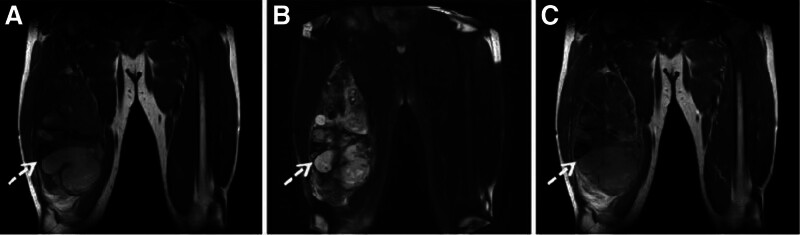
MRI revealed a huge space-occupying lesion with cystic mixed components, in the middle of the right thigh region with irregular shape and a poorly defined local boundary, measured 267 × 119 × 102 mm (white dashed arrow; A, T1-weighted coronal: T1 hypointense mass located over the middle of the right thigh region; B: T2-weighted coronal: T2 hypersignal and hypointense mixed; C: enhanced T1-weighted coronal: heterogeneous enhancement of the mass). MRI = magnetic resonance imaging.
